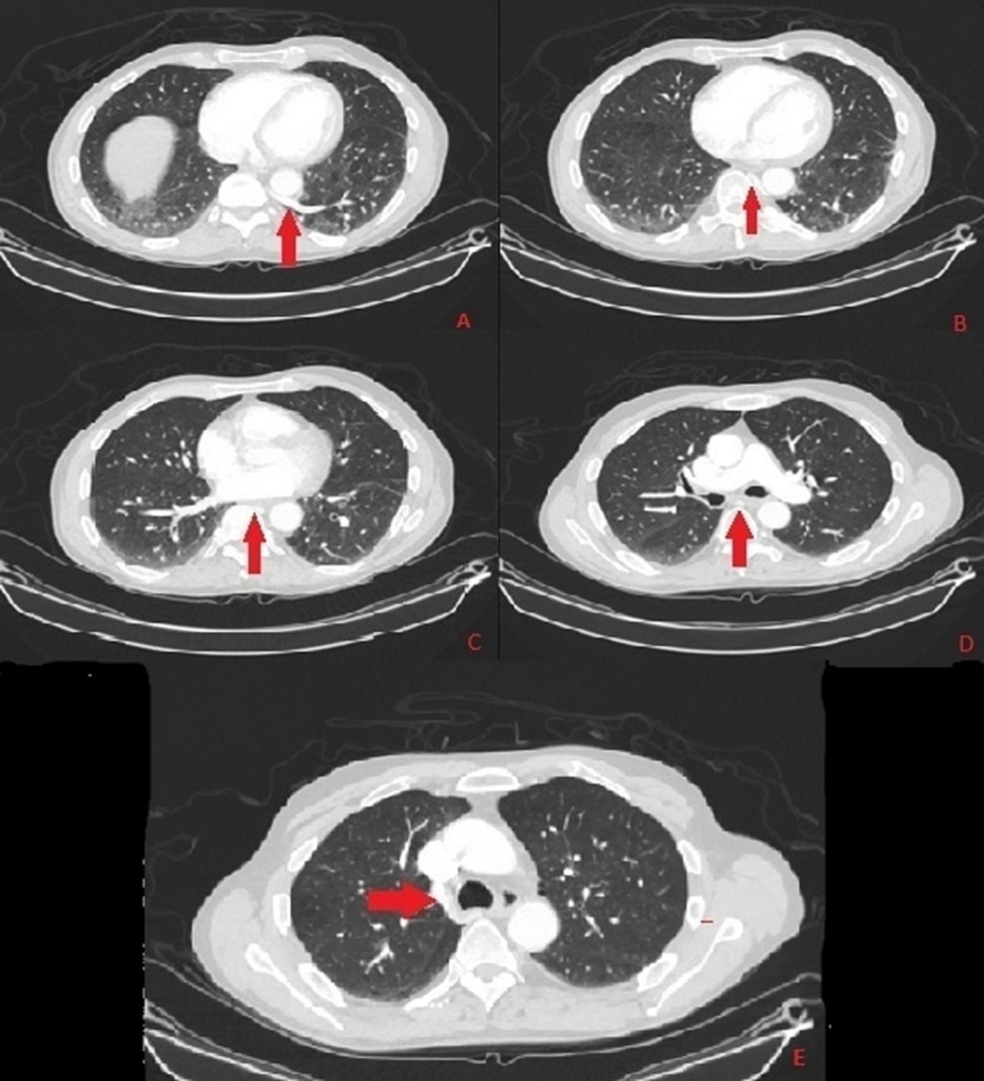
Coronal CT scan of the chest with contrast in lung window with red arrow demonstrating the course of anomalous pulmonary vein arising from intralobar sequestration (Image A) coursing on the side of the aorta (Images B, C, and D) ultimately joining azygos vein (Image E)
